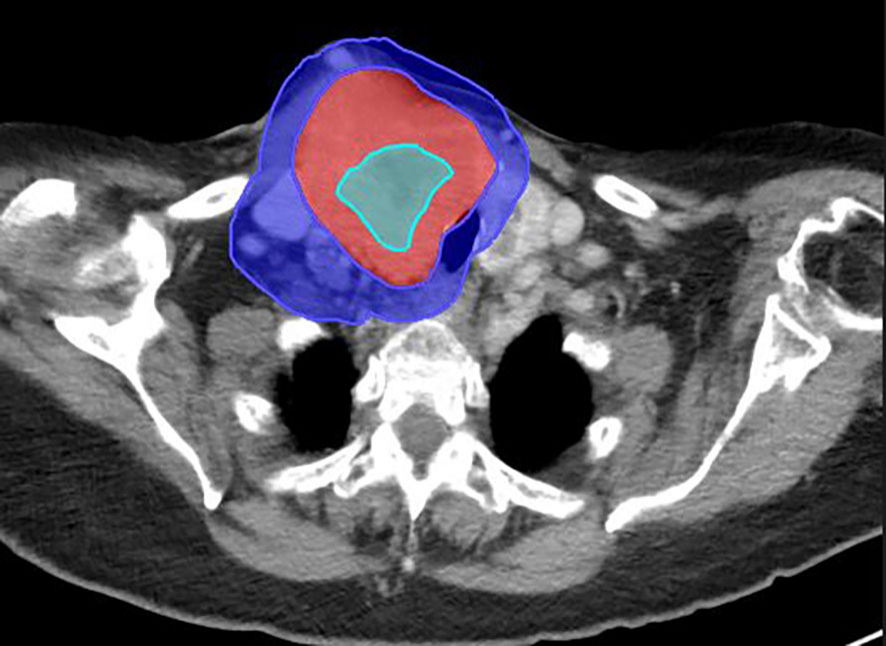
Large right neck mass from metastatic follicular thyroid cancer on the diagnostic CT scan. Mass measures 6.9 cm × 8.5 cm × 10.5 cm. The BTV (sky blue), GTV (red), and PIM (blue) are shown on this diagnostic scan.
